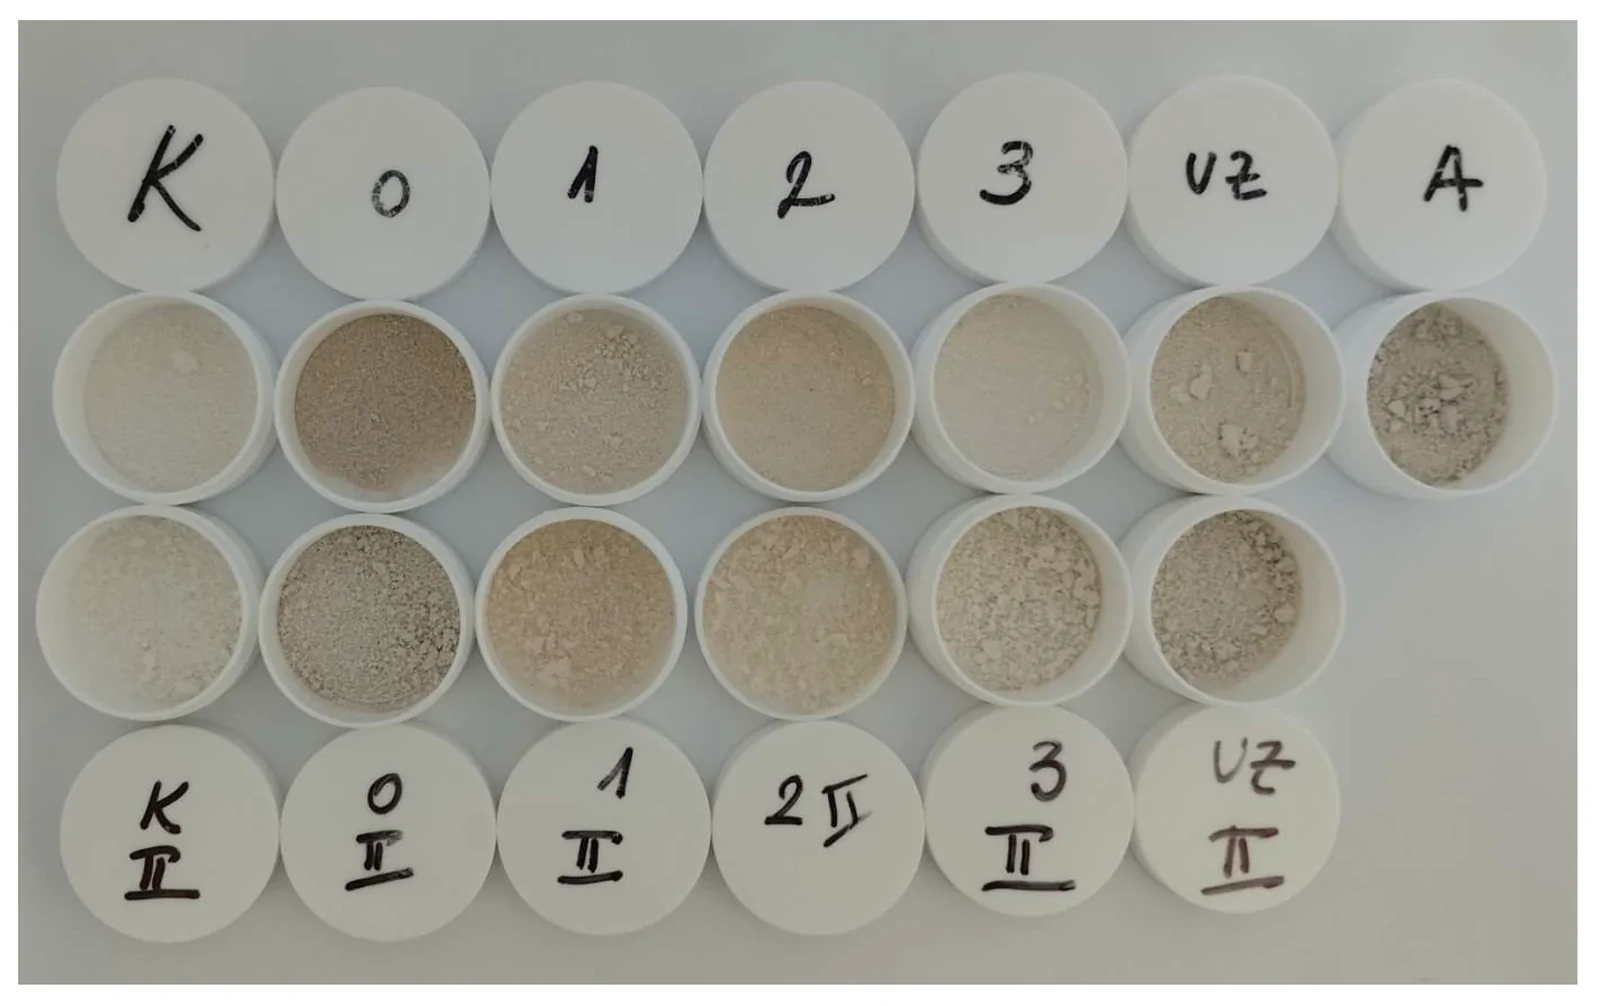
Visual appearance of obtained chitosans. 0, 1, 2 and 3 represent samples demineralized to pH 1.3 and subjected to 0, 1, 2 or 3 deproteinization baths, respectively. K denotes the sample additionally subjected to depigmentation, A the autolysis-treated sample, and UZ the ultrasound-assisted deproteinized sample. Samples marked with the suffix “II” (0II, 1II, 2II, 3II, KII and UZII) were demineralized to pH 0.5 before the corresponding deproteinization treatment.
